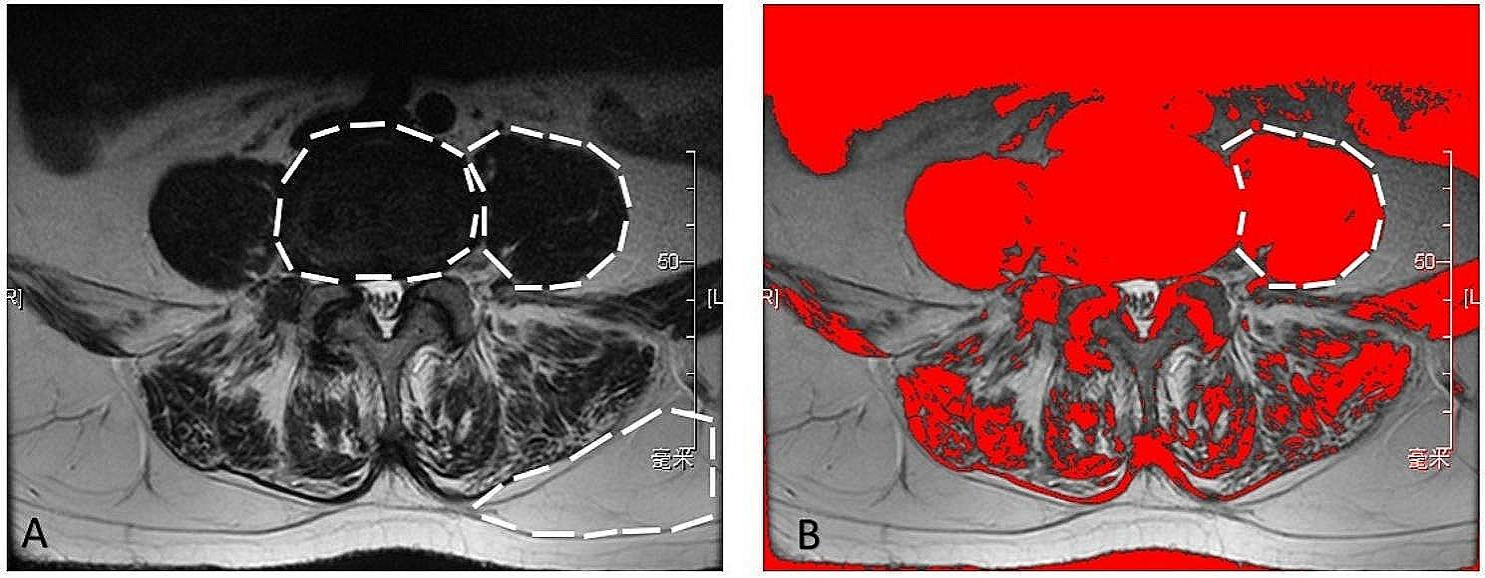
Measurements of paraspinal muscle parameters on magnetic resonance imaging. The regions of the intervertebral disc, psoas major muscle (PS), and subcutaneous fat are outlined by white lines. For the psoas major muscle, the total and functional muscles are outlined on the right and left sides, respectively. The subcutaneous fat is outlined on the left side by black lines. rtCSA = tCSA/ disc area (at the same level). rfCSA = fCSA/ disc area (at the same level). changed rtCSA = postoperative rtCSA/preoperative rtCSA. changed rfCSA = postoperative rfCSA/preoperative rfCSA. FI=(tCSA-fCSA)/tCSA. MFI = muscle index/ subcutaneous fat index. Changed rtCSA and rfCSA > 1 meant CSA increased after surgery and changed rtCSA and rfCSA < 1 meant CSA decreased after surgery
